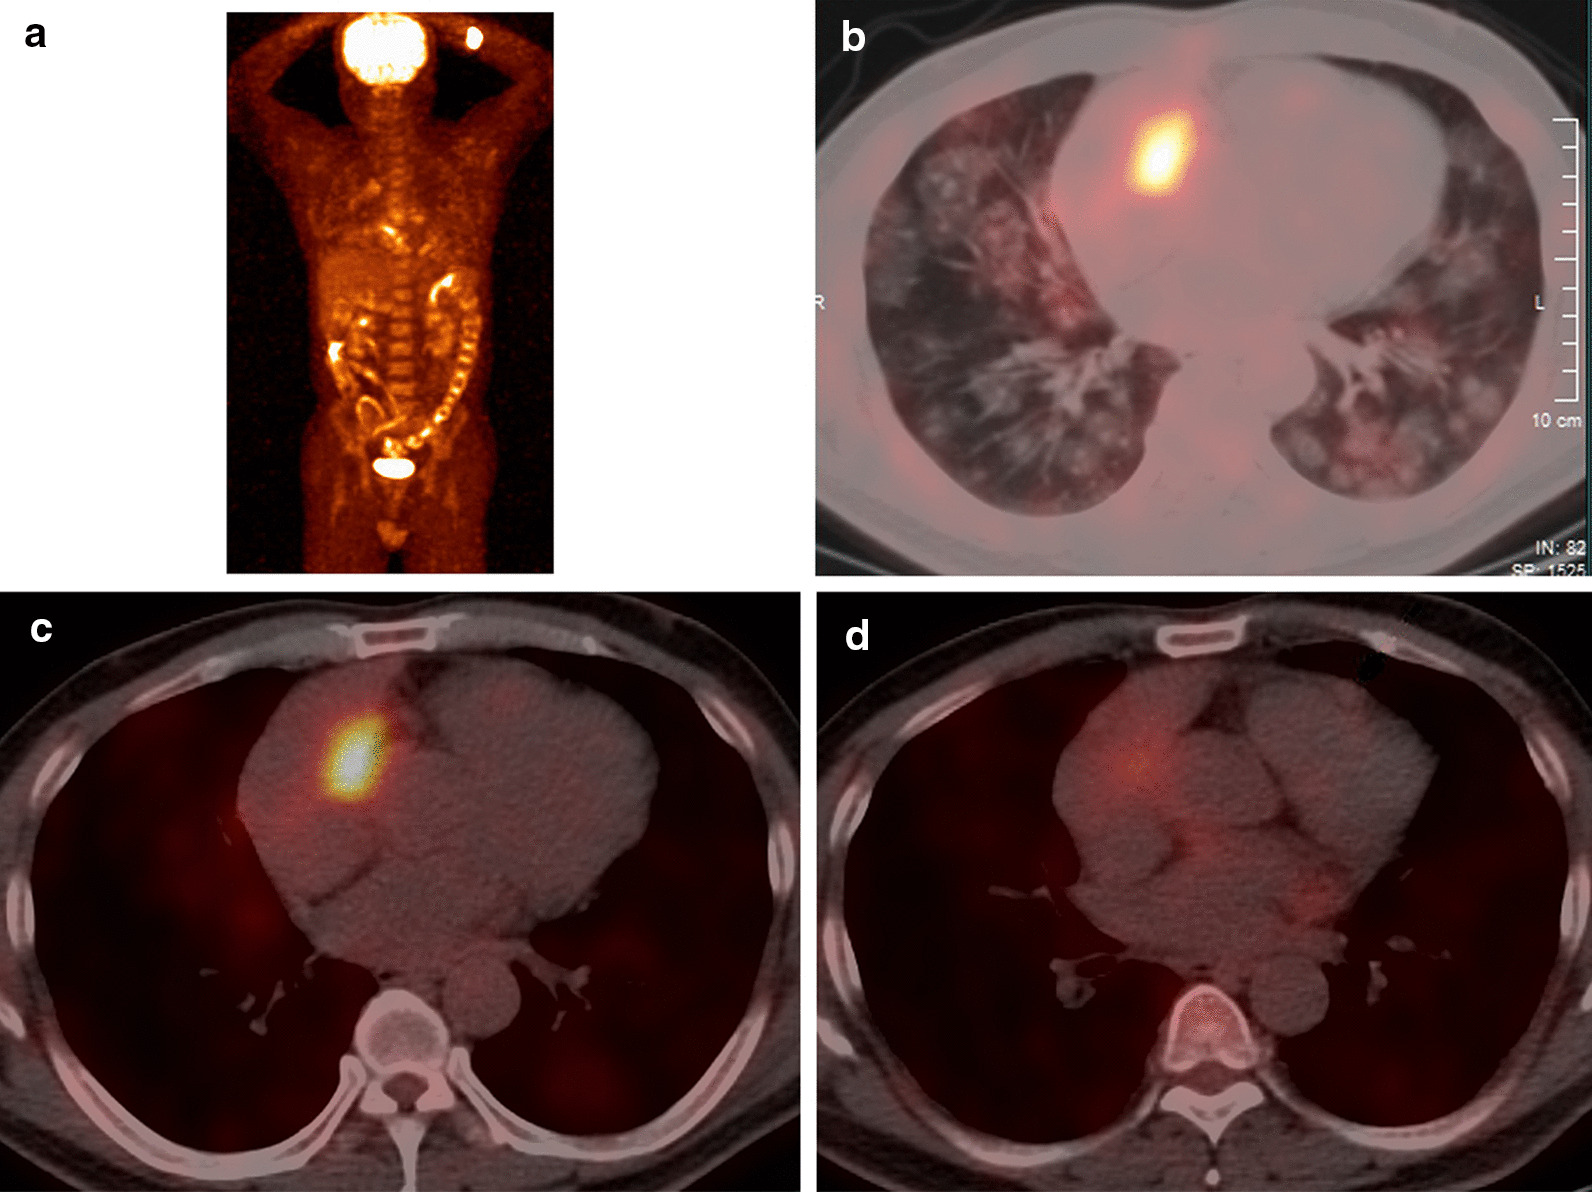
^18^F-FDG PET/CT. a The whole-body scan showed lesions limited to heart and lungs, b, c the chest scan showed increasing FDG uptake within the atrial lesion, lung nodules, d and metastatic pericardial nodules
